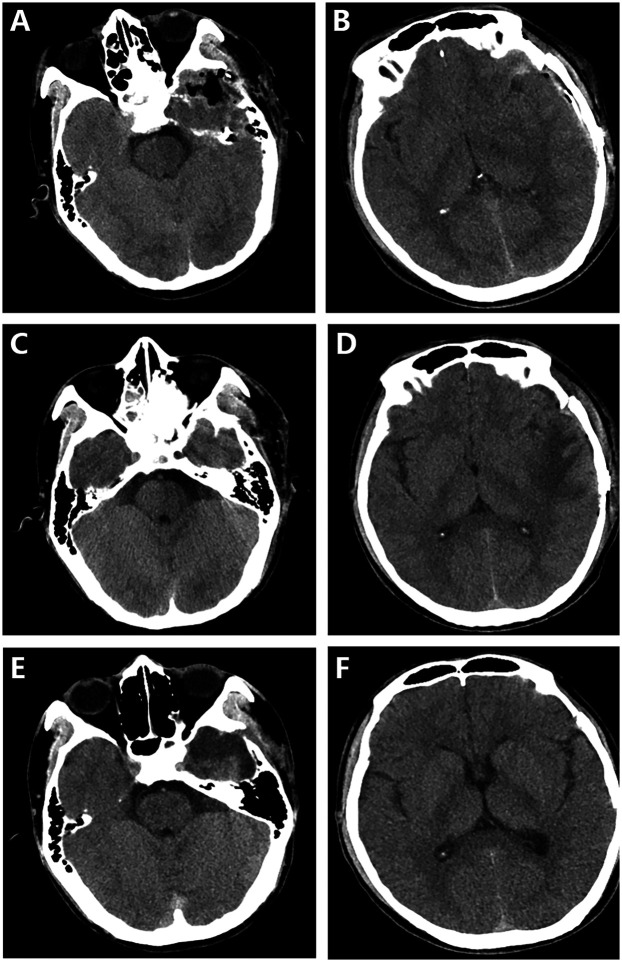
Postoperative computed tomography (CT) scans were conducted at different time points: 6 h (A,B), 1 week (C,D), and 1 month (E,F) after the surgery. The follow-up CT images clearly demonstrated the successful removal of both the foreign body and the hematoma, as well as satisfactory neurological recovery.
